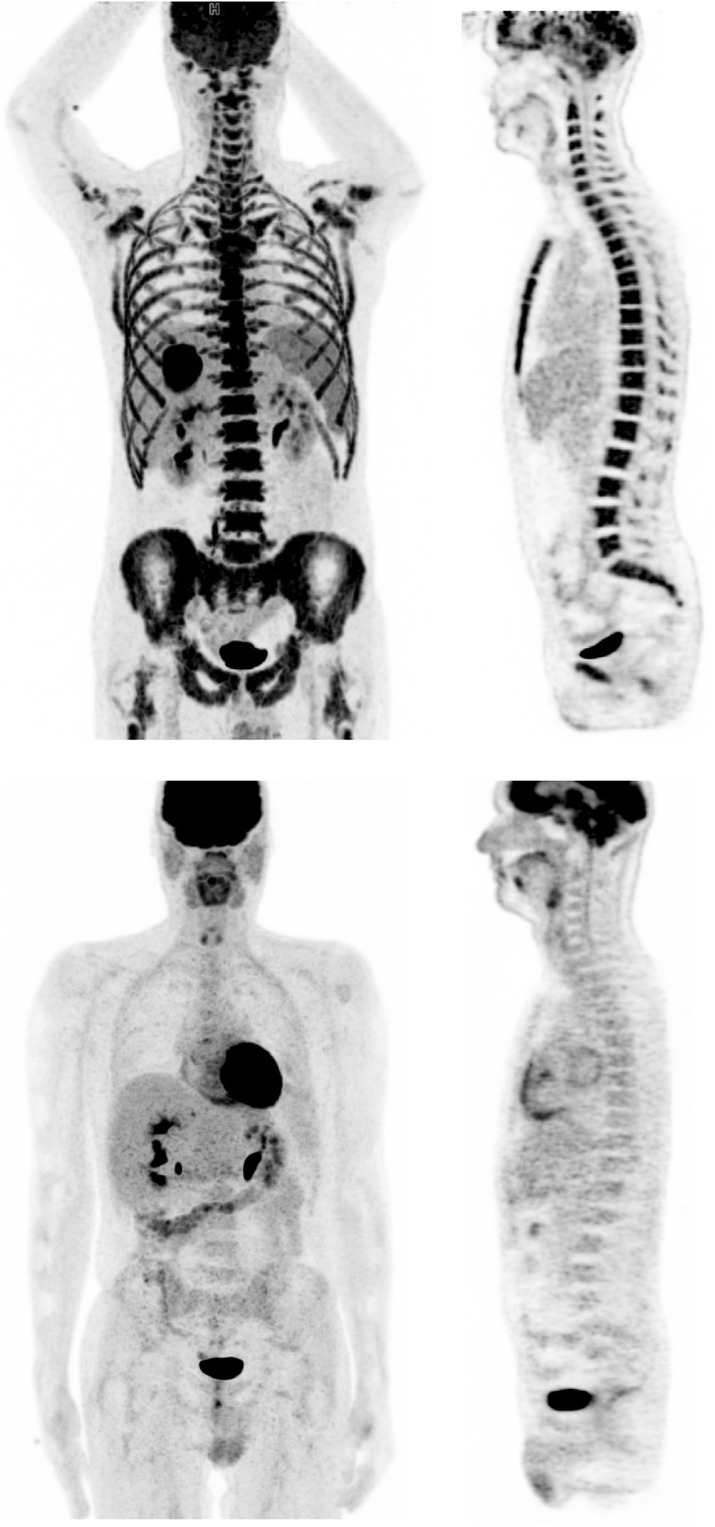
FDG PET tracer uptake before (upper row) and after (lower row) surgery: after resection of the tumor FDG PET showed complete normalization of bone marrow metabolism
